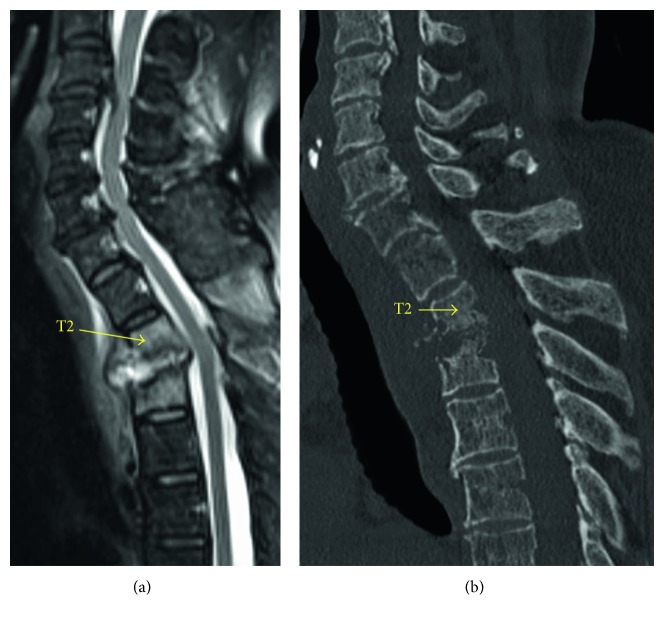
(a) Preoperative sagittal T2-weighted magnetic resonance image of the cervicothoracic spine shows vertebral osteomyelitis and intervertebral disk abscess of T2/3. (b) Preoperative sagittal CT shows bone destructions of the endplates of T2/3.
